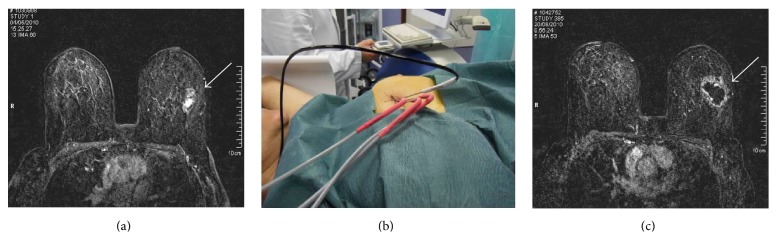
A 50-year-old woman was diagnosed as having invasive ductal carcinoma and distant metastases. Axial contrast-enhanced T1-weighted fat suppression MR image showed the primary breast cancer located in the external quadrant of the left breast measuring 27 mm in its major diameter (arrow) (a). She was submitted to CT-guided cryoablation of the breast tumor under local anesthesia and conscious sedation. We used three cryoprobes along with one thermocouple for temperature monitoring (b). Axial contrast-enhanced T1-weighted fat suppression image 2 months after the procedure showed complete ablation with a large nonenhanced area, due to tissue necrosis, surrounded by a ring of enhanced tissue compatible with granulation tissue in the proliferative phase (arrow) (c).
